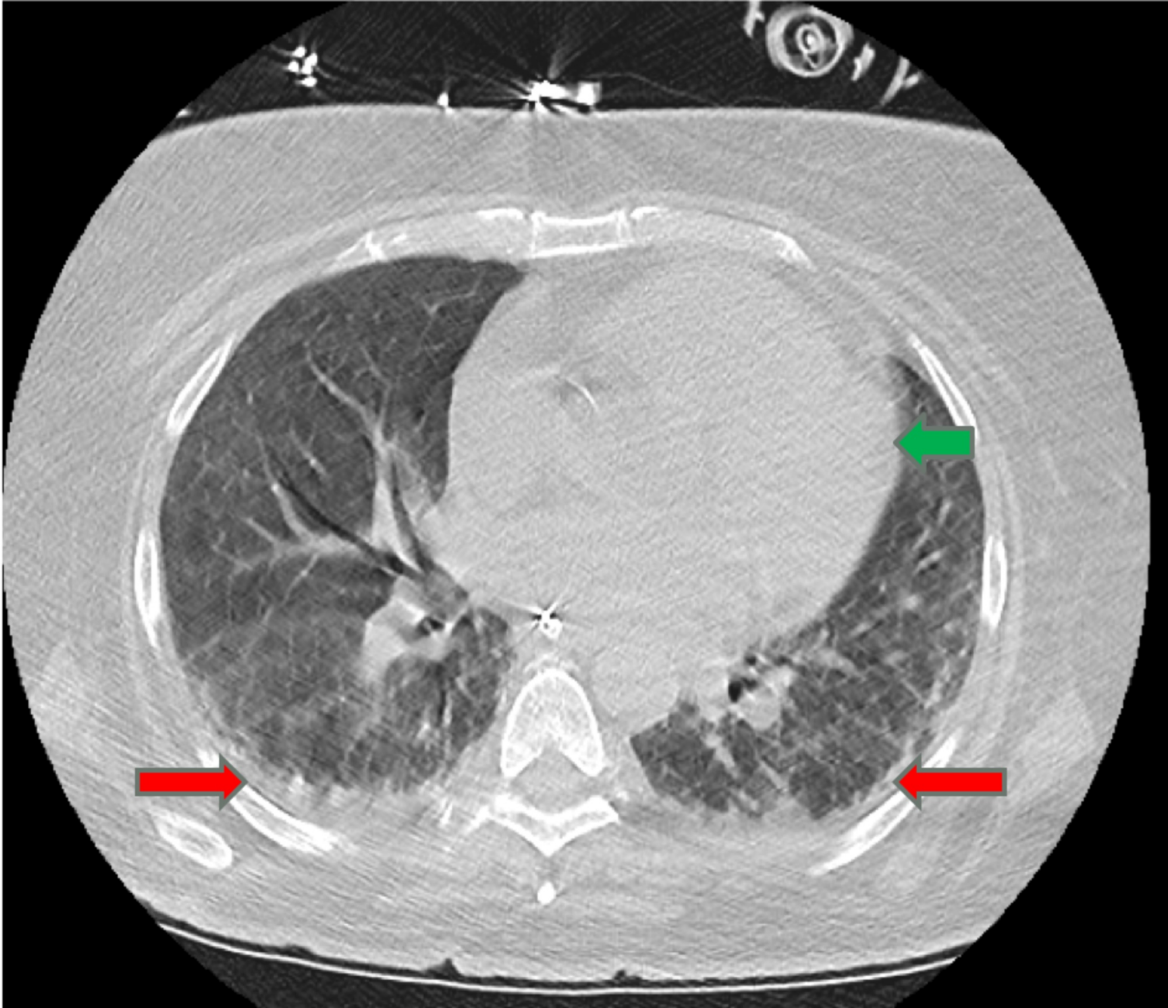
Chest CT Bibasilar atelectatic changes (red arrow), no gross evidence of interstitial lung disease. Cardiomegaly (green arrow).
